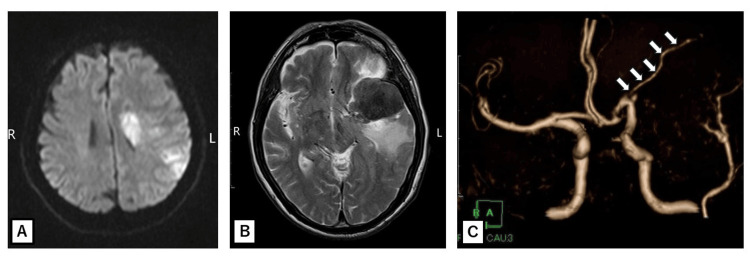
Initial brain imaging findings (A) Diffusion-weighted image demonstrating acute infarction in the left territory of the middle cerebral artery, which appeared as a hyperintense signal due to restricted diffusion. (B) A T2-weighted image revealing a well-demarcated, hypointense extra-axial mass along the left sphenoid ridge, which is consistent with meningioma, with surrounding edema involving the adjacent frontal and temporal lobes. (C) Time-of-flight magnetic resonance angiography showing complete occlusion of the proximal segment of the left middle cerebral artery. The arrows indicate the recurrent artery of Heubner.
